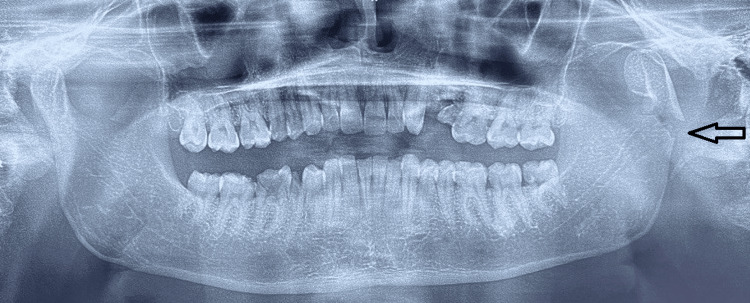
OPG revealing a left condylar neck fracture OPG: Orthopantomogram The arrow shows the left condylar neck fracture
